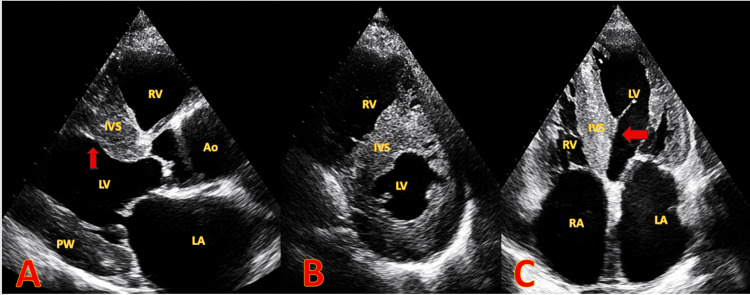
Transthoracic echocardiographic features suggestive of CA Transthoracic echocardiography demonstrating typical morphological features of CA. (A) Parasternal long-axis view showing marked left ventricular wall thickening and a granular sparkling appearance of the interventricular septum (red arrow). (B) Parasternal short-axis view demonstrating concentric left ventricular hypertrophy. (C) Apical four-chamber view showing biventricular hypertrophy with biatrial enlargement and marked thickening of the interventricular septum (red arrow). Ao: aorta, IVS: interventricular septum, LA: left atrium, LV: left ventricle, PW: posterior wall, RA: right atrium, RV: right ventricle, CA: cardiac amyloidosis
